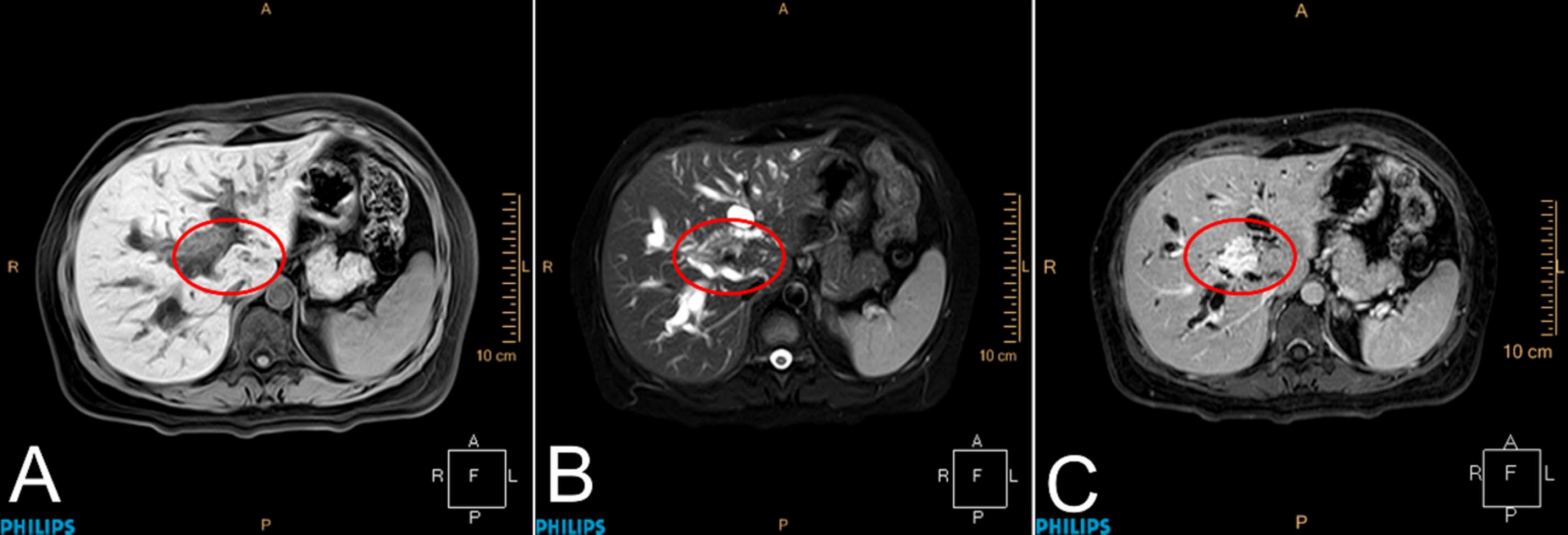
Magnetic resonance imaging of the liver. Patchy abnormal signals were observed at the confluence of the left and right hepatic ducts in the hilar region of the liver, with a maximum cross-sectional area of approximately 2.0 × 1.4 cm. Enhanced scanning showed gradual and uniform enhancement. The lesion was distributed along the bile duct, and the distal bile duct wall was slightly thickened and enhanced. The proximal bile ducts were significantly dilated. The lesion was adjacent to the main left and right branches of the portal vein. Portal lymph nodes were visible. (A) T1WI, (B) T2WI, and (C) DCE-T1, marked with a red circle.
